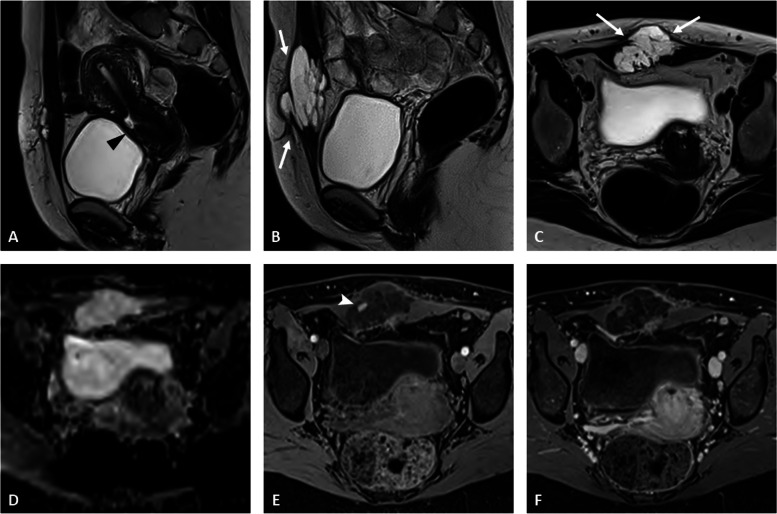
Clear cell adenocarcinoma of the abdominal wall in a 44-year-old woman with a history of cesarean section. Sagittal T2-weighted sequence (a) shows the scar on the anterior uterine isthmus (black arrowhead). Sagittal (b) and axial T2-weighted (c) images show a multiloculated cystic lesion developed within the anterior abdominal wall (arrows), infiltrating the right rectus abdominis muscle, without obvious diffusion restriction (d). The axial fat-suppressed T1-weighted image shows a small hemorrhagic spot (arrowhead) within the cyst (e). Diffuse enhancement of the cystic cavity wall and internal septae is seen on fat suppressed-enhanced T1 image (f). There was no surgery-associated pelvic endometriosis in this patient.
